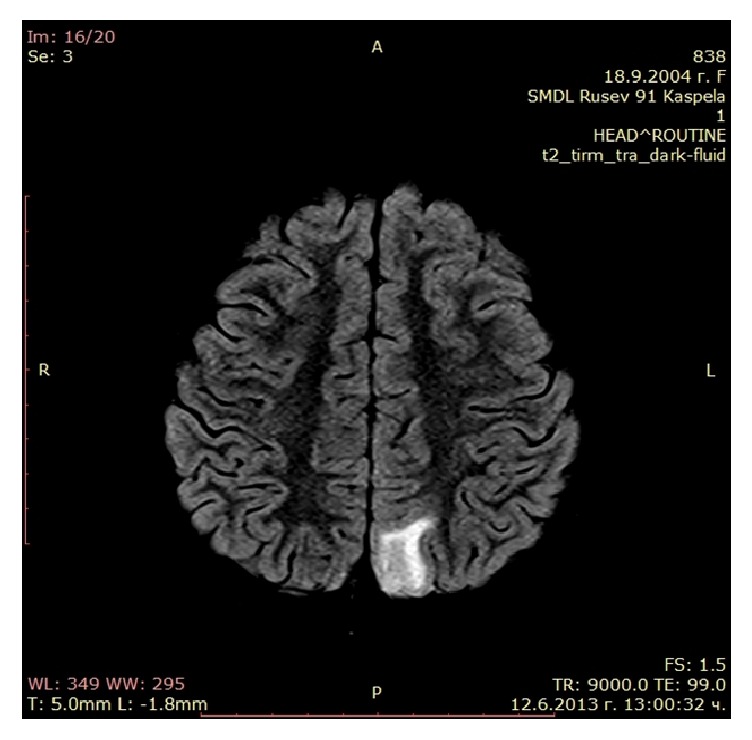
Cerebral МRI: hyperintense in Т2 and FLAIR lesion in the left occipital area.
